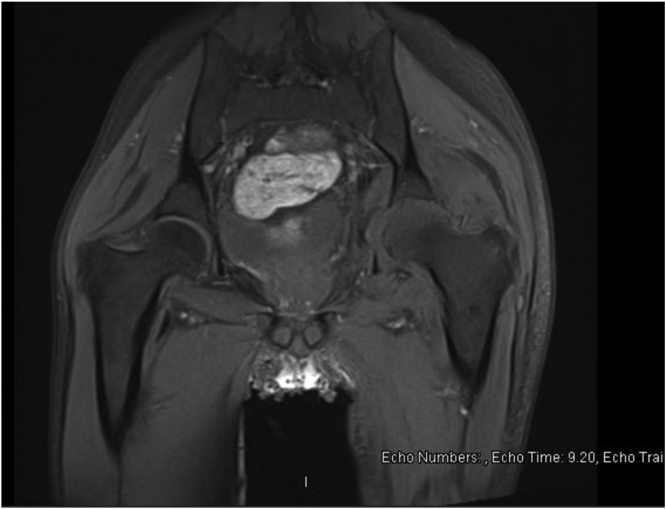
Follow up MRI of the left hip showing appreciable interval decrease in the synovial thickening and inflammatory changes involving the surrounding muscles.
